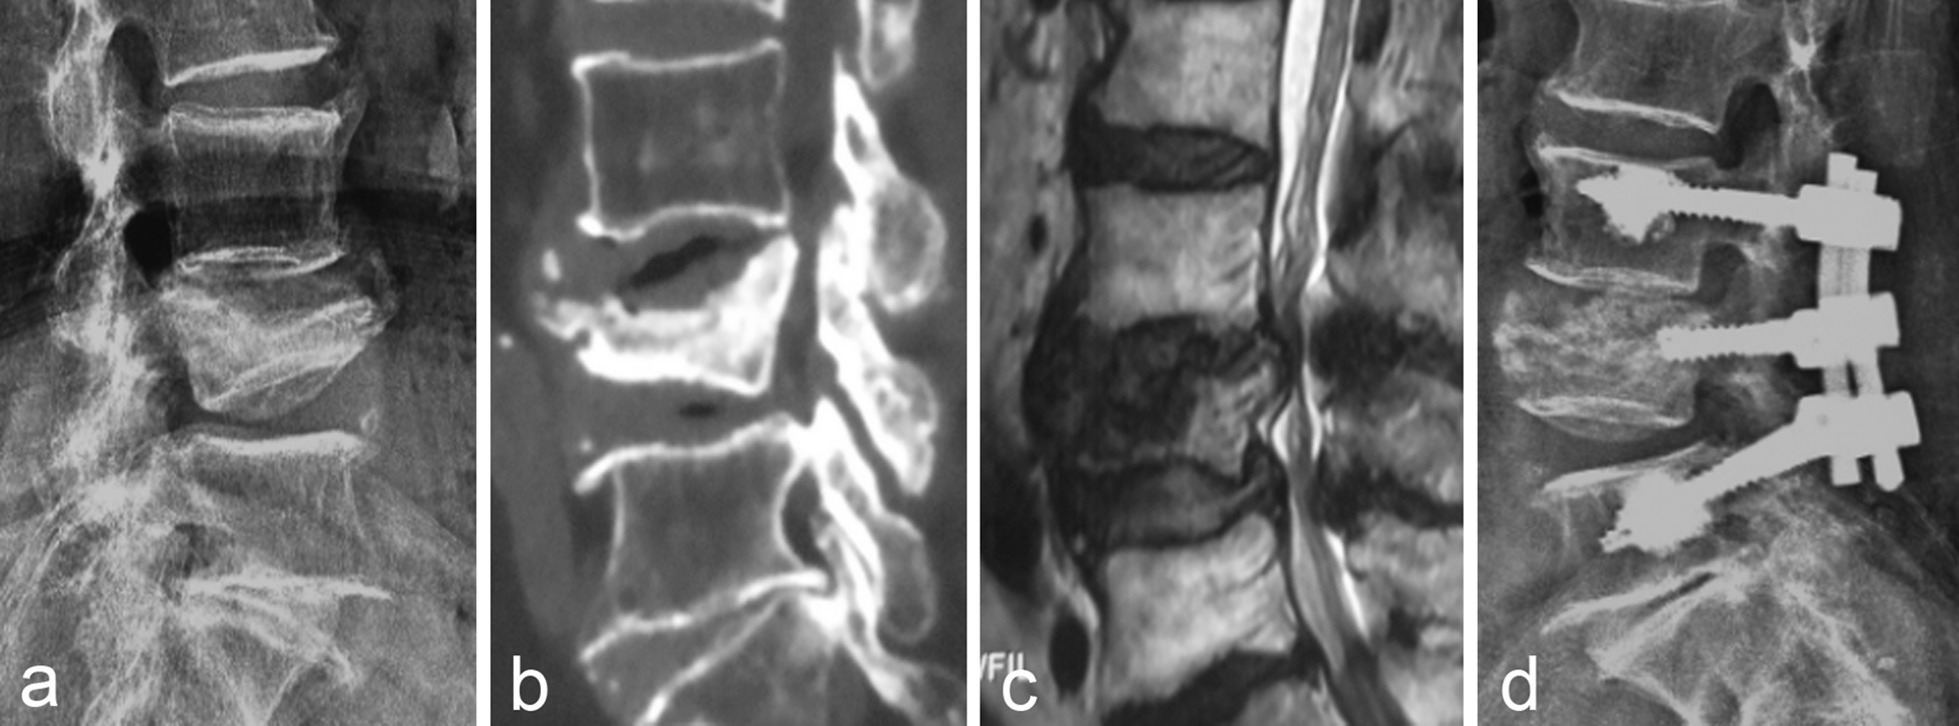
A 73-year-old female patient (Grade III) presented with mild back pain due to trauma 4 months ago, and presented with increased pain and numbness and weakness in both lower limbs 1 month ago, accompanied by intermittent claudication. a Lumbar spine X-ray showed the height of the L4 vertebra was lost and the endplate collapsed; b Sagittal CT showed collapse of the L4 vertebral body, low-density shadow in the vertebral space, protrusion of fracture block into the spinal canal, and spinal stenosis at the same level; c MRI T2 showed abnormally low signal in the L4 vertebra, with obvious dural compression and spinal stenosis at the same level; d Postoperative X-ray showed that the patient underwent posterior decompression and bone graft fusion with cement-reinforced internal fixation
